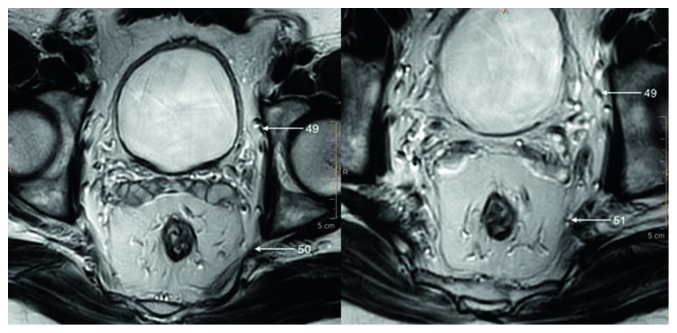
T2-weighted axial image of regional nerves.
